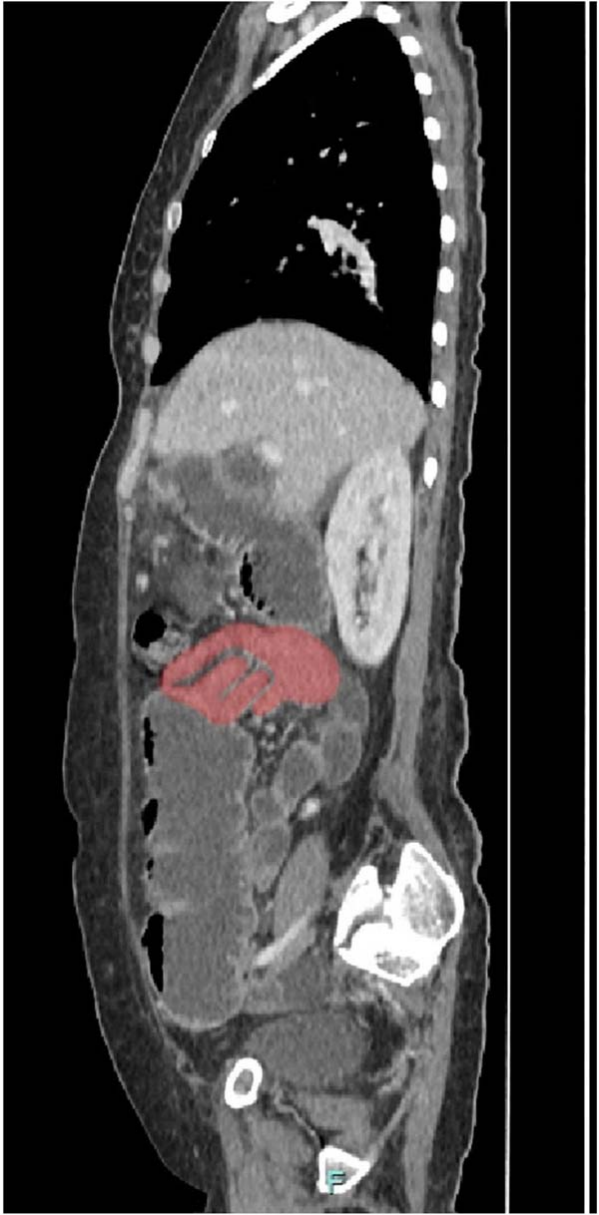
Sagittal view of CT scan. The involved bowel loops are thickened with mesenteric invagination into the intussusception. The distal small and large bowel appears collapsed.
